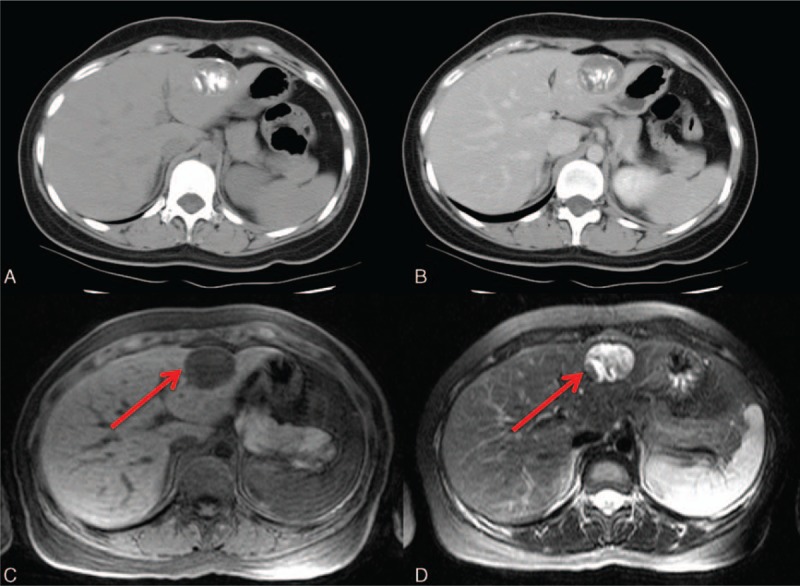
A 24-year-old woman with Echinococcus infection. A, Partially calcified hydatid cyst is well depicted on the precontrast CT image. The calcification is a consequence of the host–antigen reaction. B, The cystic component is without enhancement. C, Typical hypointensity on the T1-weighted image. D, Typical hyperintensity on the T2-weighted image. Note the characteristic hypointense rim on both the T1- and T2-weighted images (*arrows* in (C) and (D)), indicating the collagen produced by the host. This is the “frontline” of the host and the antigen.
